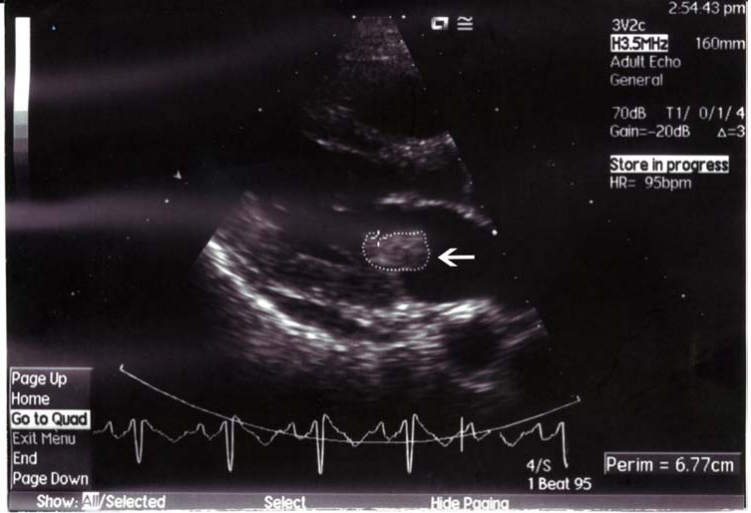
Transoesophageal echocardiogram showing perforation of the posterior mitral cusp with significant mitral regurgitation (spontaneous echo contrast in left atrium).
